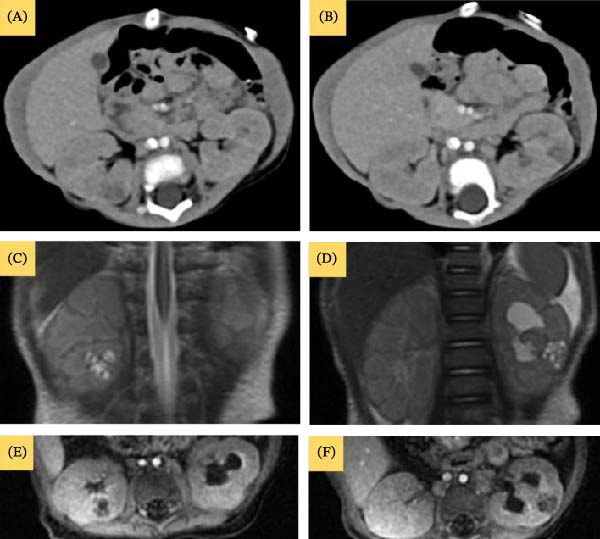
(A, B) Enhanced axial CT scan of the abdomen at the level of kidneys showing bilateral interpolar complex cystic solid lesions. (C, D) T2WI showing bilateral multiseptated cystic lesions. (E, F) T1 postcontrast and coned‐down view of the kidneys shows early homogeneous septa and periphery enhancement. Left moderate hydronephrosis is also appreciated (Case 1).
